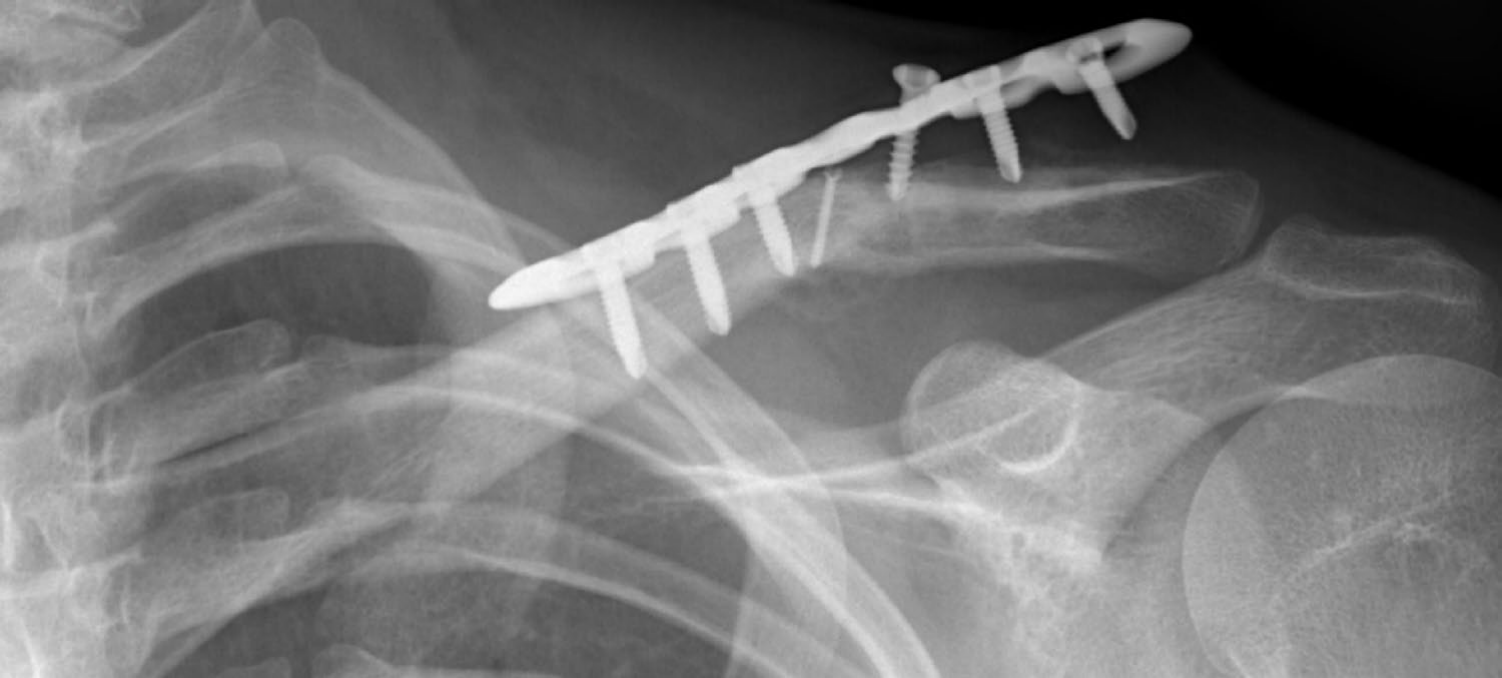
A cut-out of the screws in the lateral fracture fragment like shown in the x-ray can occur in respect to the demanding high forces acting on the lateral end of the clavicle
